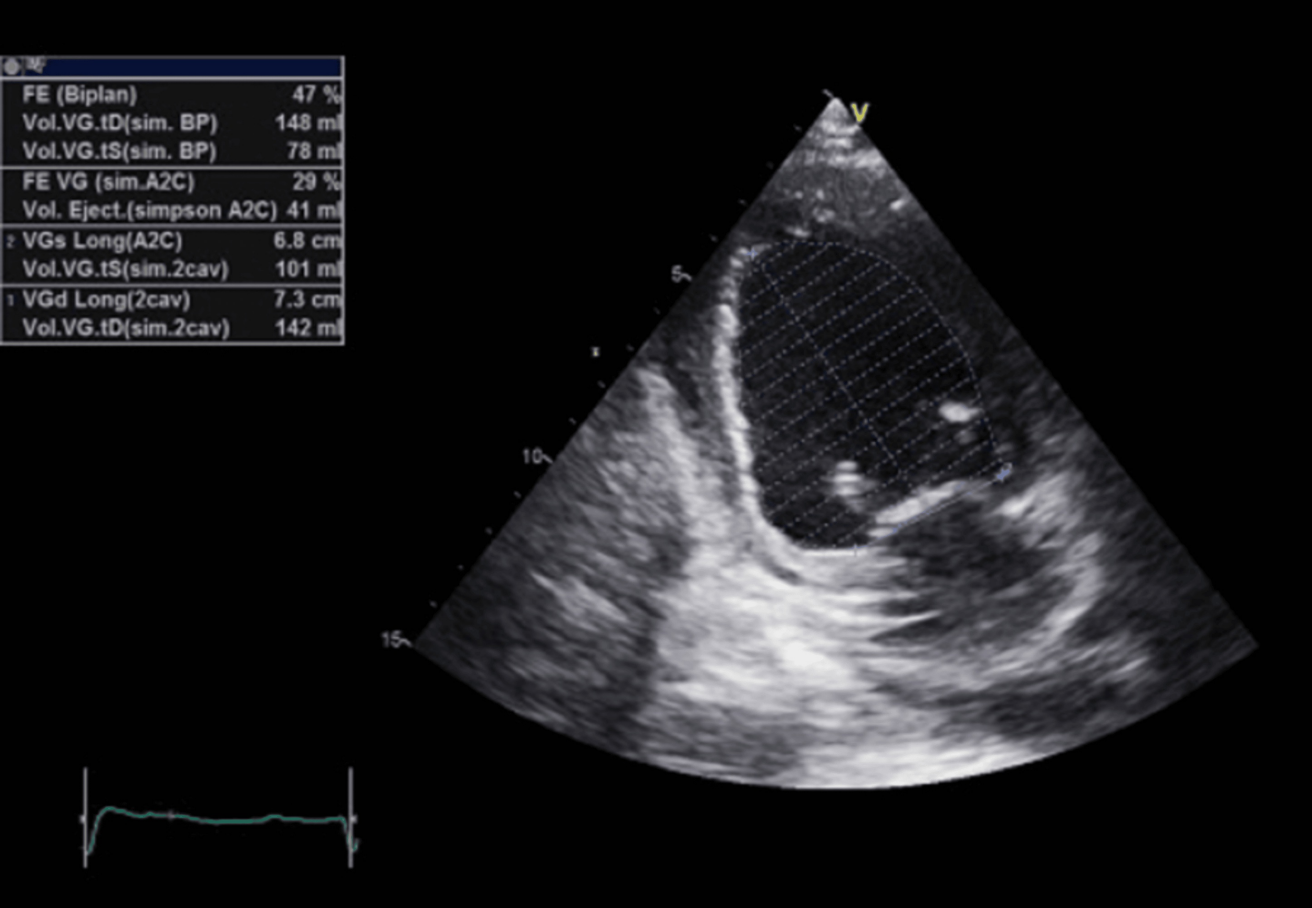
Transthoracic echocardiography A transthoracic echocardiography two-chamber shows moderately impaired systolic function and an ejection fraction of 47%.
